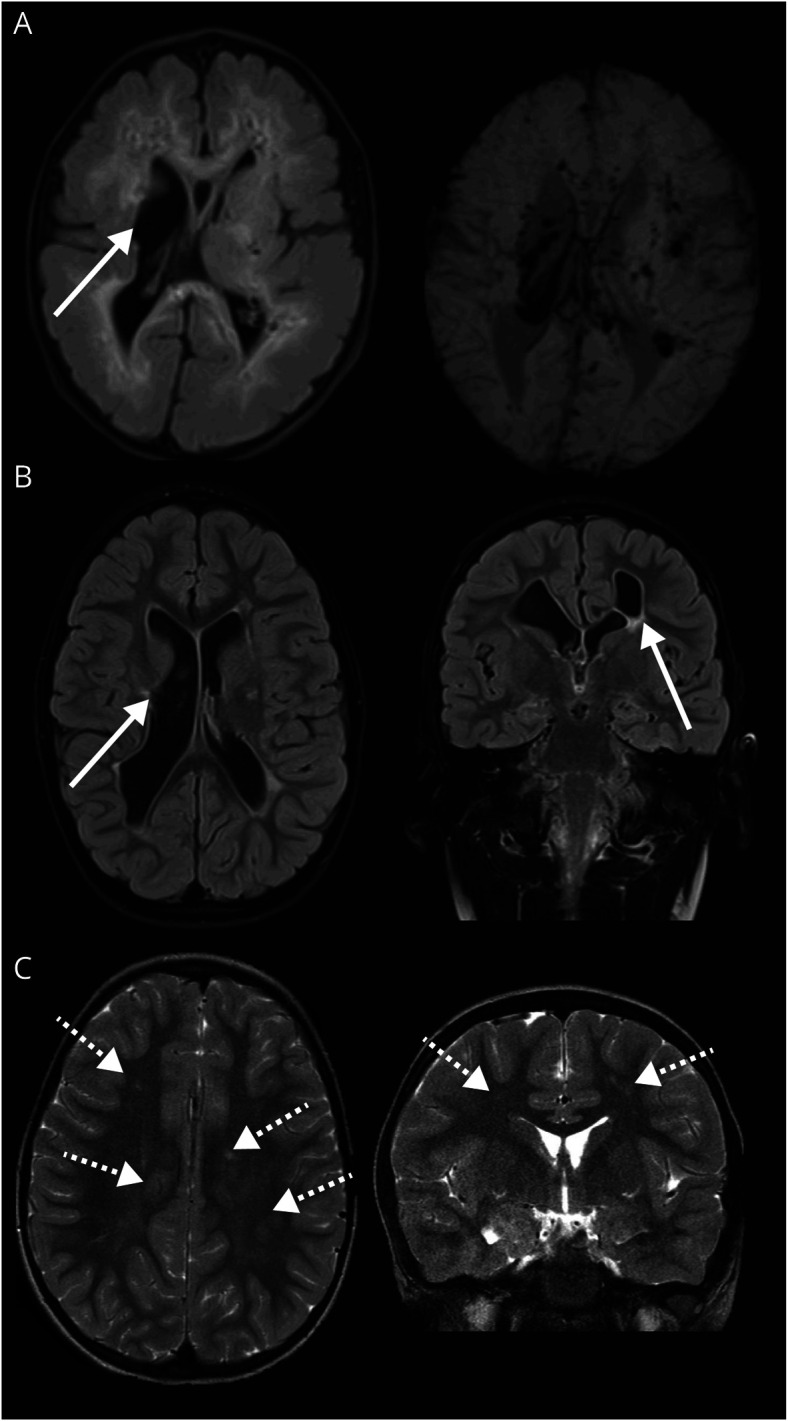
Neuroimaging Patterns in *COL4A2*-Related Disorder (A) Porencephaly with basal ganglia involvement and PVHI pattern (thick white arrow) in a patient with *COL4A2* variant with perinatal presentation, imaged in first 2 weeks of life (axial T2/FLAIR, GRE), (B) porencephaly with basal ganglia involvement and PVHI pattern (thick white arrows) in patient with *COL4A2* variant with early childhood presentation (axial and coronal T2/FLAIR), and (C) nonspecific small foci of hyperintense signal on T2WI in subcortical white matter in a patient with *COL4A2* variant with late childhood presentation (axial and coronal T2) (dashed thick arrows). Each panel represents a different patient. FLAIR = fluid-attenuated inversion recovery; GRE = gradient echo; PVHI = periventricular hemorrhagic infarction; T2WI = T2-weighted imaging.
